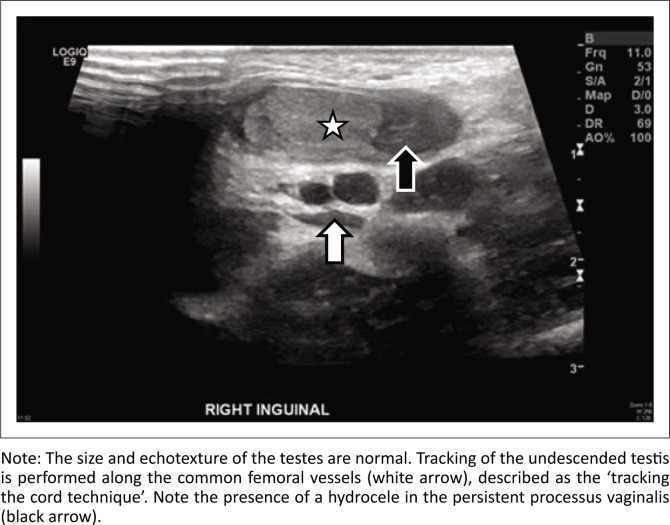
Greyscale ultrasound in a 6-month-old infant showing an undescended testis in the right inguinal canal (star).
